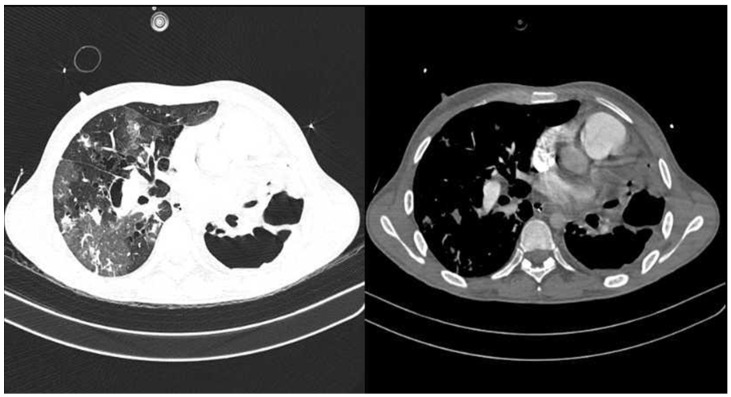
Chest angiotomography on admission. Axial contrast-enhanced chest CT images showing extensive bilateral pulmonary involvement with multiple cavitary lesions predominantly in the right lung, associated with areas of consolidation and parenchymal destruction, consistent with severe infectious processes.
